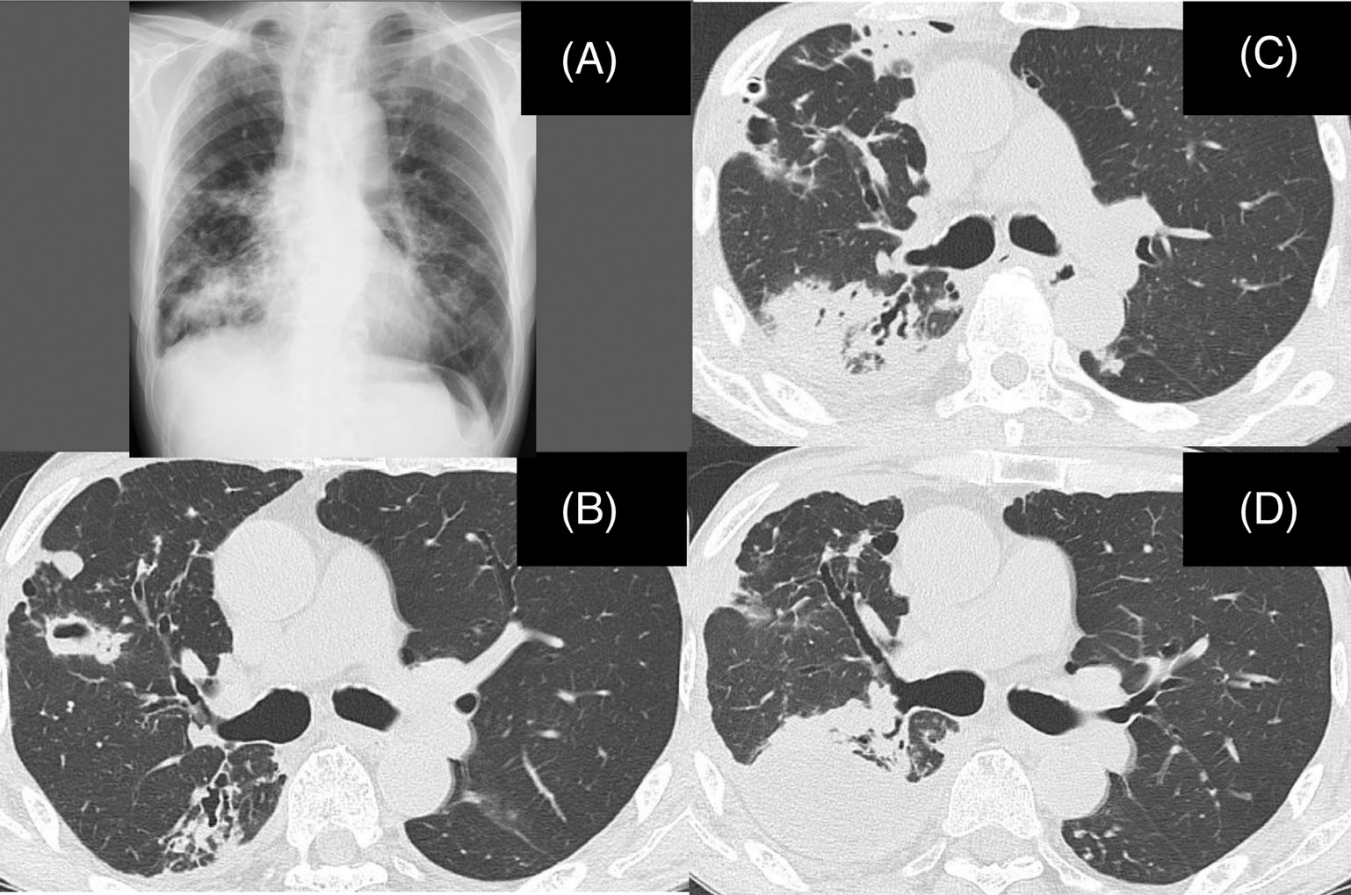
Chest x‐ray revealed consolidation in the right lower lung field and multiple nodules in the bilateral lung fields at 1 year before admission (A). Chest computed tomography (CT) revealed bronchiectasis and multiple small cavities and nodules in both lungs at 1 year before admission (B). Chest CT on Day 7 post‐admission revealed consolidation in the right upper lobe (C). The consolidation in the right upper lobe has decreased, although pleural effusion due to the effects of pleurodesis was observed at 3 months after discharge from hospital (D).
